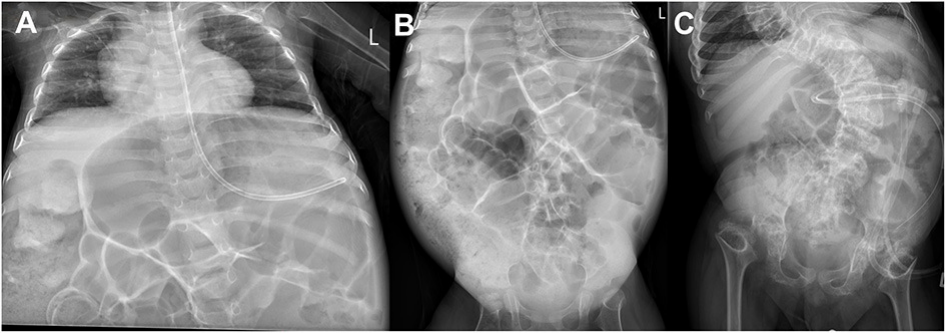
Abdominal X-rays of patients 1 and 3. (A,B) Patient 3 at age 2 years; severe dilatation of the stomach and bowel loops and fecal impaction especially in the right colon. (C) Patient 1 at age 8 years; a similar stomach and bowel loop dilatation.
